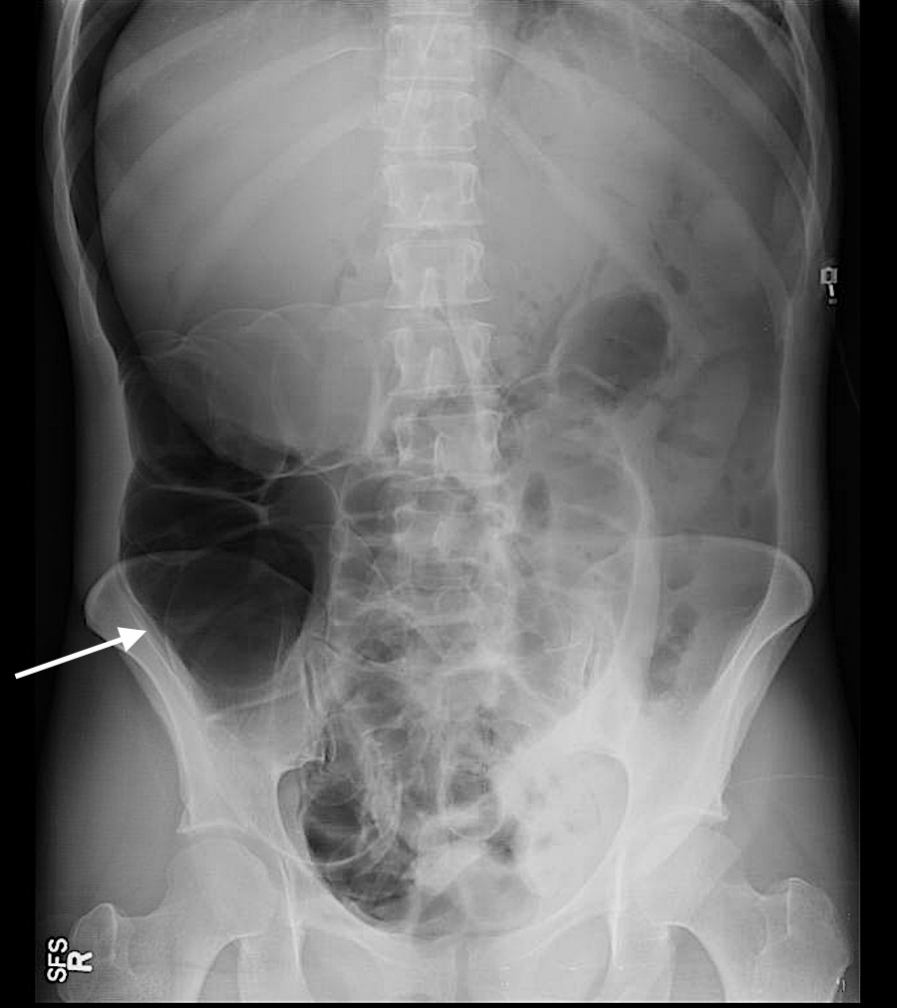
X-Ray abdomen A significant amount of free air is seen within the abdomen. Given the patient's history of recent colonoscopy and acute abdominal pain, this likely represented intestinal perforation.
